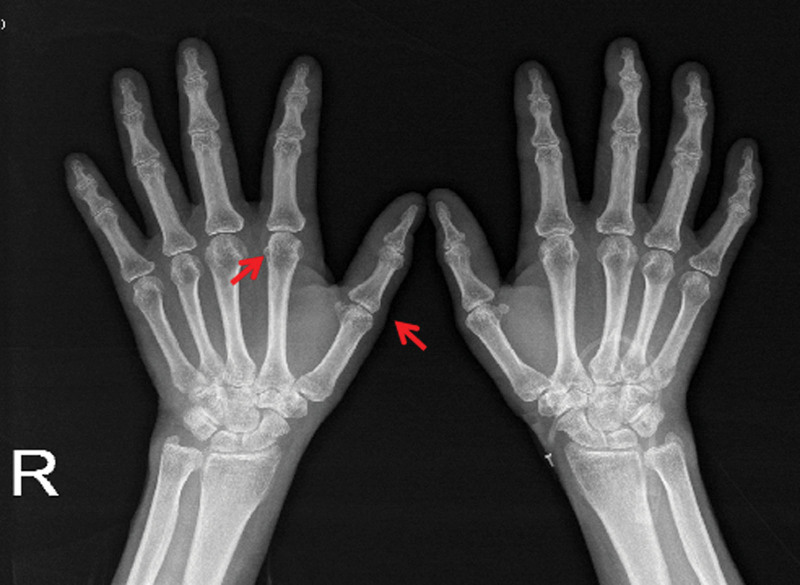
X-ray images of patient hands. Arrows showed osteoporosis of both hands; soft tissue swelling around the proximal interphalangeal joints of both hands and the first metacarpophalangeal joint of the right hand.
